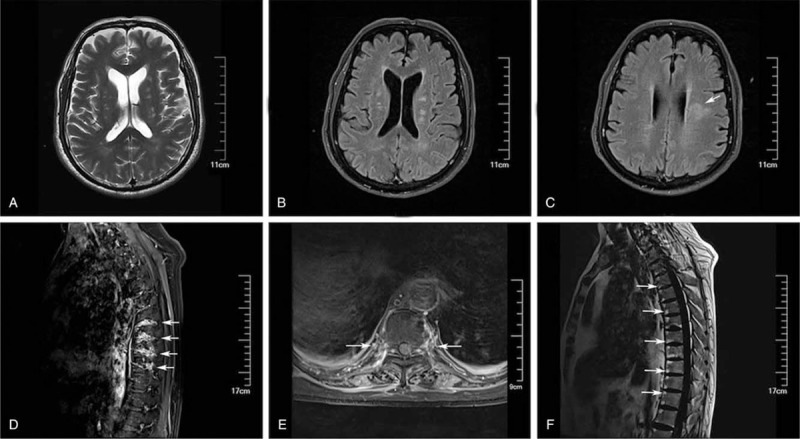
An MRI series of the brain and spine of the patient. Figure panels depict the following findings: (A) a brain MRI revealed T2 hyperintense lesions in the brainstem; (B) a brain MRI revealed multifocal T2 hyperintense lesions within cerebral white matter; (C) a corresponding fluid attenuated inversion recovery (FLAIR) sequence shows hyperintense signals in cerebral white matter; (D) a sagittal T1 post-contrast fat saturated Dixon sequence of the spine revealed enhancement of thoracic nerve roots; (E) a corresponding axial MRI of thoracic spine; and (F) a spinal T_1_-weighted MRI shows multiple spondylitis of anterior corners. MRI = magnetic resonance imaging.
